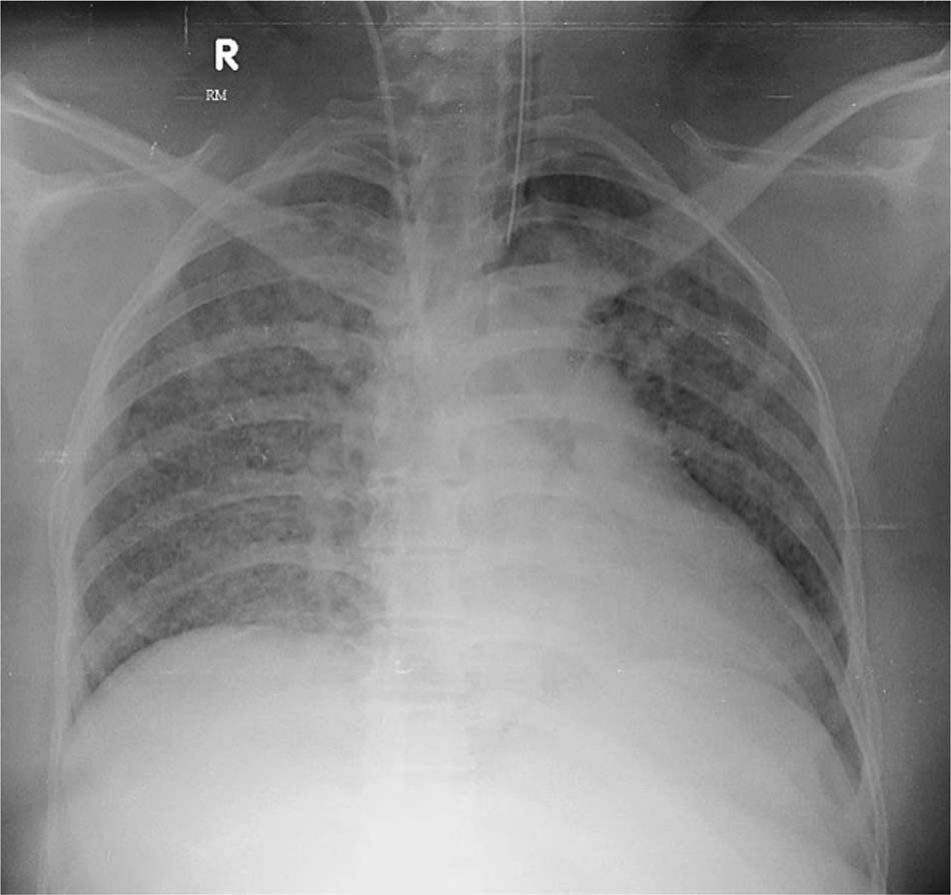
Chest radiograph showing progressive miliary mottling.
